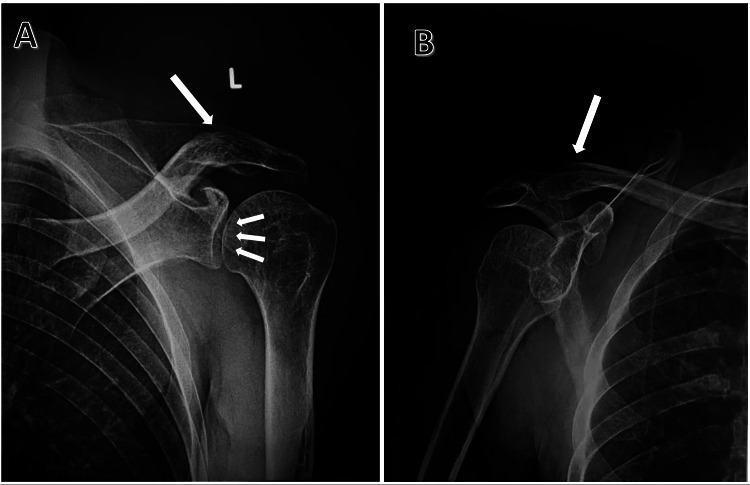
(A) Anteroposterior and (B) lateral X-rays of the left shoulder showing the stable alignment of the glenohumeral joint and the consolidation of the outer portion of the clavicle fracture.
